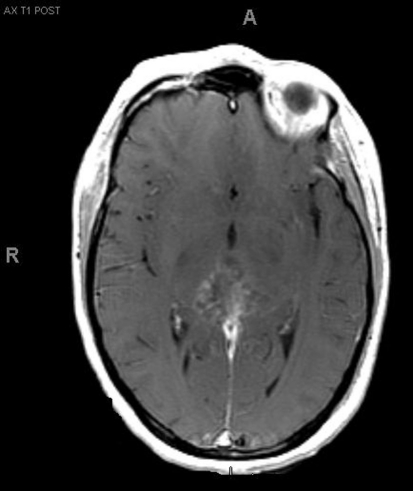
MRI after second resection.
